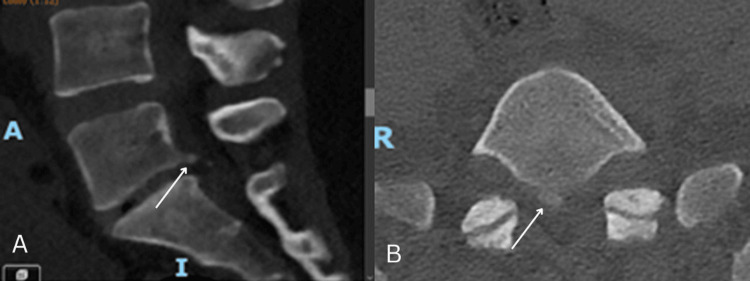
CT scan of the lumbar spine: (A) sagittal and (B) axial reconstructions. Detachment of the posterior and inferior margins of the L5 vertebra indicated by the arrow.
